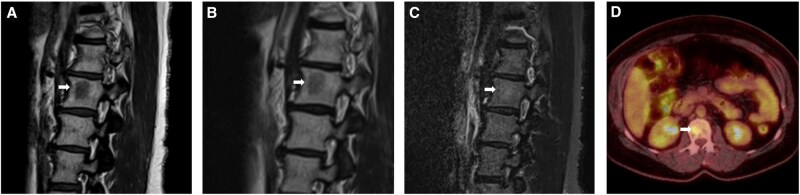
Abnormal L2 vertebral body lesion (white arrow) in a 56-year-old female with a history of breast malignancy. (A-C) sagittal MRI sequences with low T1w signal (A) and low T2w signal (B) lesion, both higher than the adjacent disc signal, and a subtle increase in STIR signal (C). (D) Axial FDG PET study demonstrates an avid lesion with a SUV of 4 within the same period (C).
